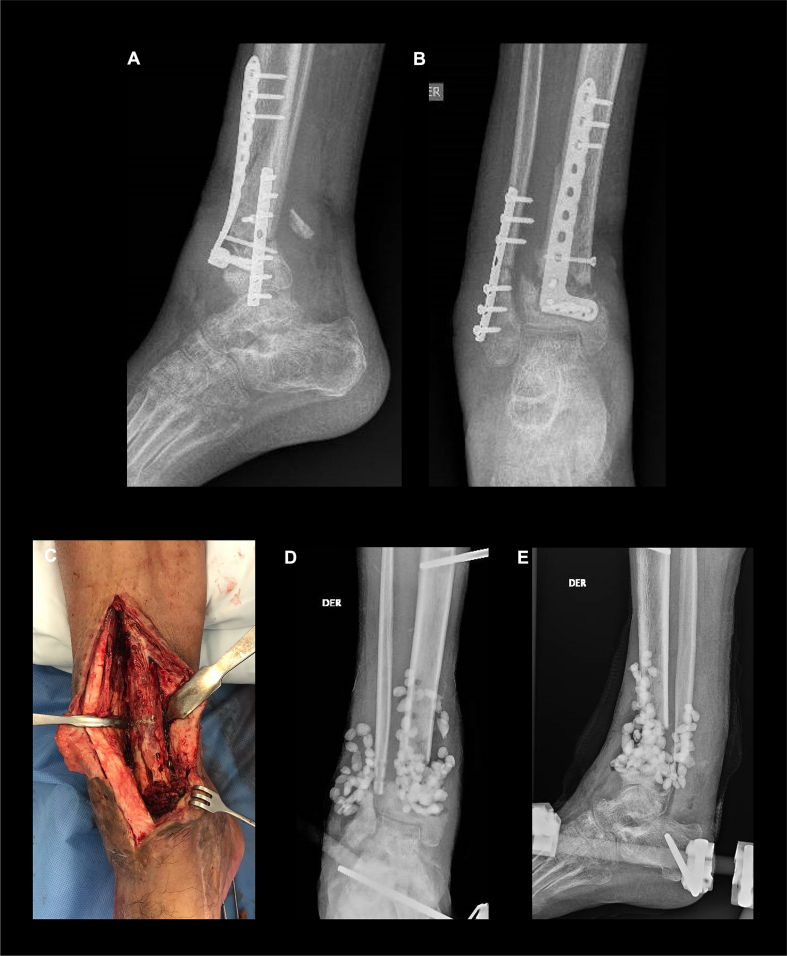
A–B): A 46-year-old man with a type IIIa open fracture in his right leg. X-rays show non-union in the distal tibia. C) X-ray showing infected soft tissue and bone. D–E) Stage 1 with resection of non-union focus and placement of antibiotic beads.
